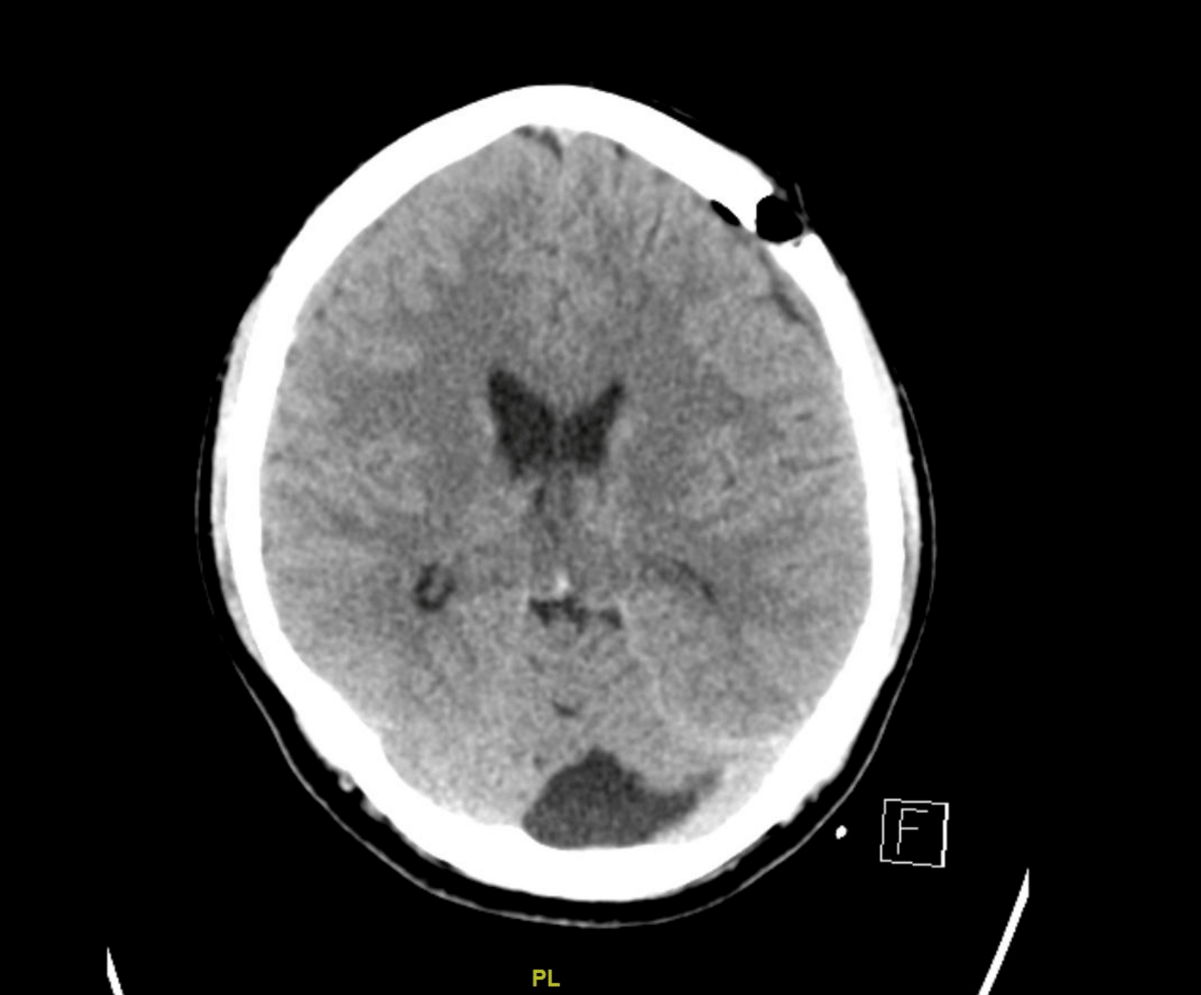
CT of the brain after burr hole drainage of the left SDH showing improvement of mass effect.
